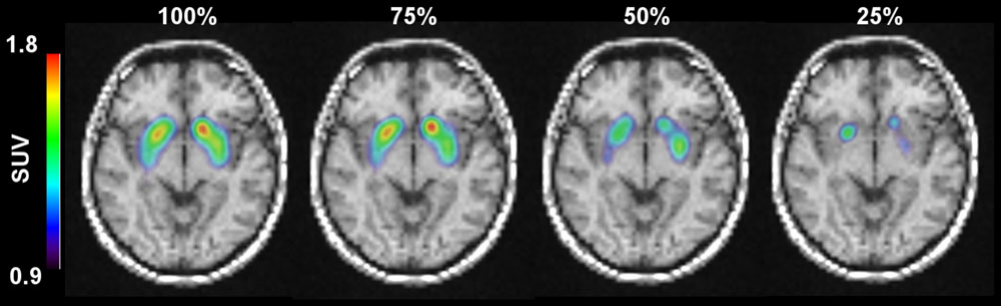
^99m^Tc‐TRODAT‐1 computed tomography (SPECT) overlays on magnetic resonance imaging (MRI) images for various datasets from one of the study subjects. The SPECT images were reconstructed using 4i10s with Butterworth post‐filtering (cutoff value = 0.4 cm^−1^, power value = 10) for better image display.
